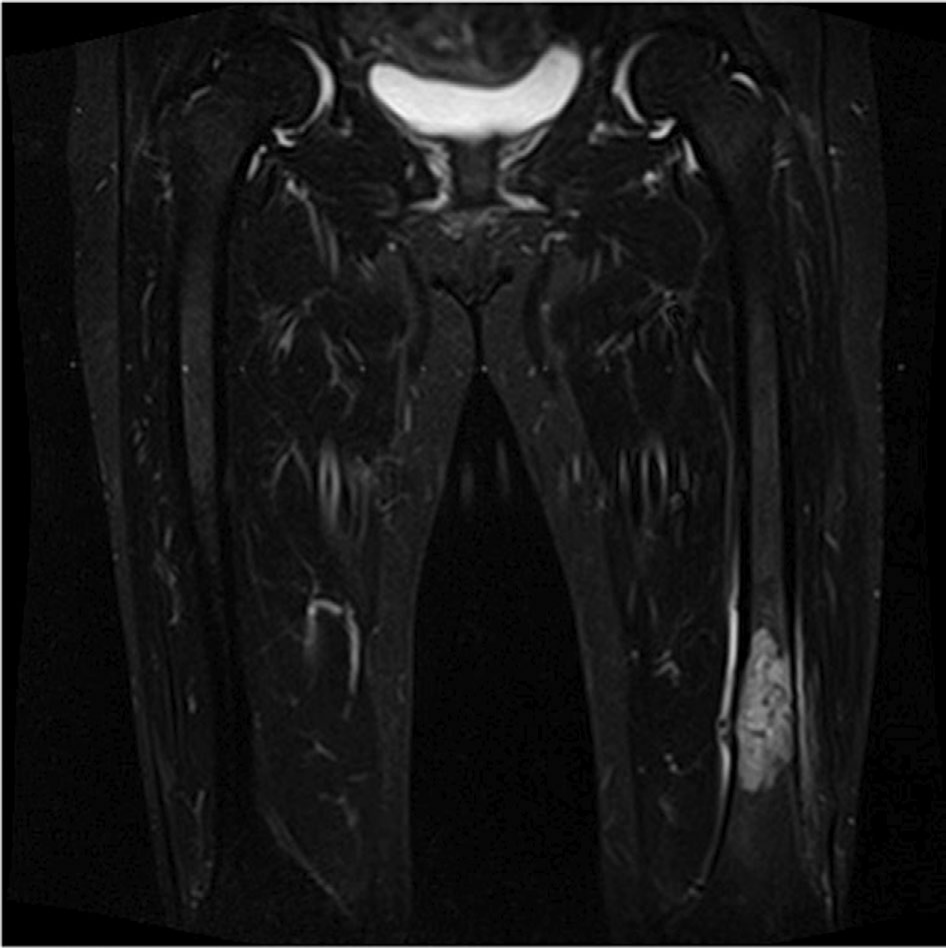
MRI showed findings of a malignant bone tumor of the left femur.
